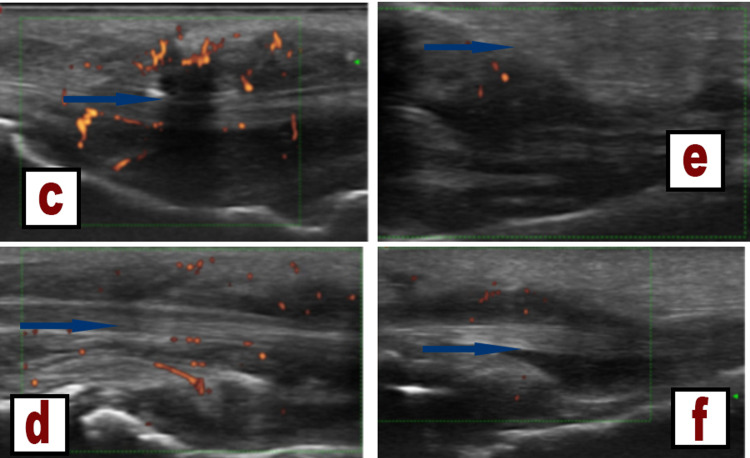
Ultrasonography of the hands and feet. (c) Ultrasonography of the hands showing grade 2 inflammatory tenosynovitis in the right flexor digitorum longus. (d) Ultrasound of the hands showing a major dactylitis of the fourth finger on the left. (e) Ultrasound of the feet showing grade 2 inflammatory tenosynovitis of the flexor 1 of the toes. (f) Ultrasound of the feet showing grade 2 inflammatory tenosynovitis of the flexor 2 of the toes.
